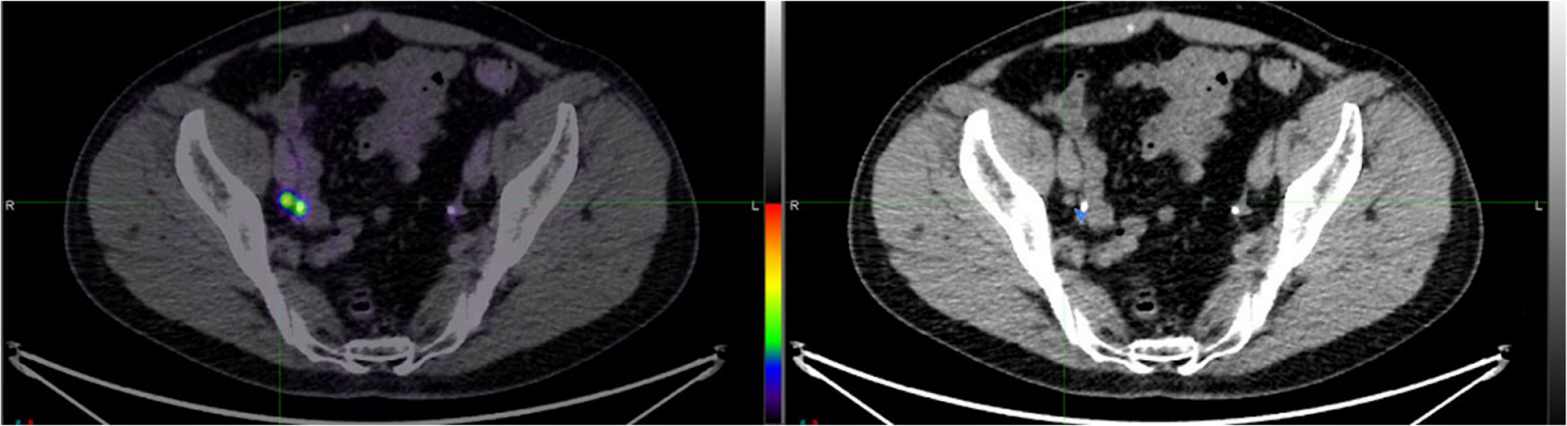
PSMA PET/CT imaging in this male with biochemical recurrence of prostate cancer demonstrates activity in the distal ureter, which is clearly identified by colocation with radiographic contrast on the fused image (left) whereas the small node lateral to this is also seen, consistent with small volume nodal metastasis
